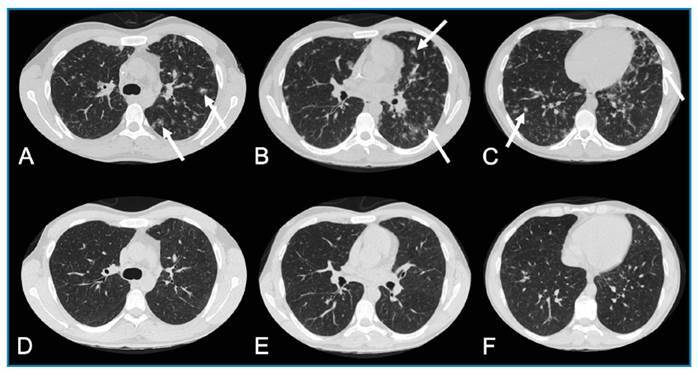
Pulmonary syphilis in a 26-year-old man. (A-C) An axial chest CT scan showing multiple nodules in both lungs, some with peripheral ground-glass halos, constituting the halo sign (arrows). (D-F) A chest CT scan performed 2 weeks after receiving penicillin G showing the resolution of all lesions.
